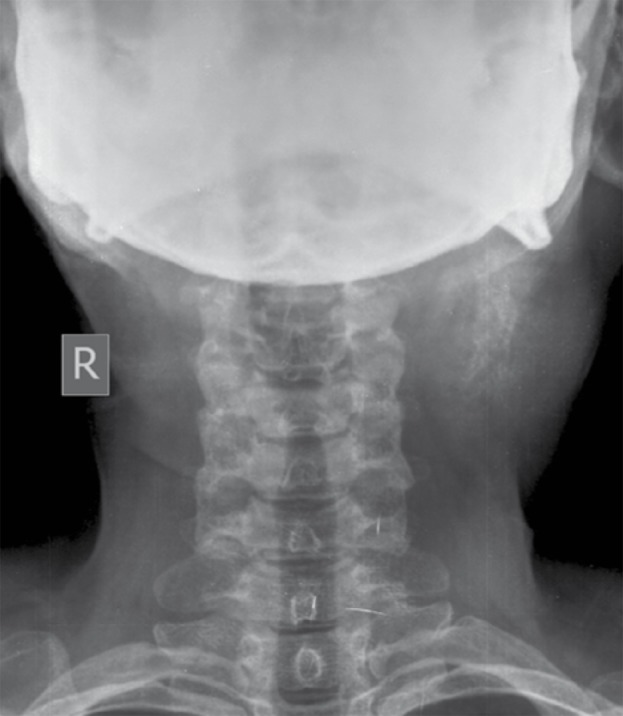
Plain Radiograph, AP View of the Neck Shows Soft Tissue Opacity on the Upper Left Side of the Neck With Central Amorphous Calcifications.
